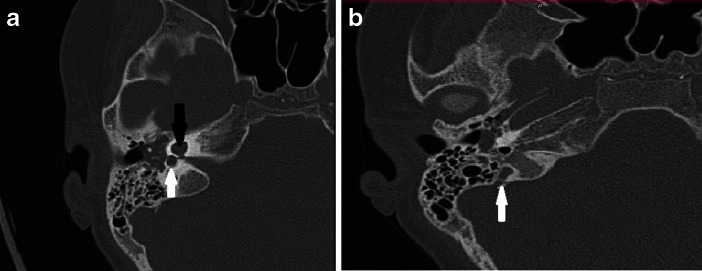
a. CT scan, temporal bone, axial view shows preserved basal turn but absence of the apical turns of the cochlea (black arrow), dilated vestibule (white arrow). b. CT scan, temporal bone, axial view shows enlarged vestibular aqueduct (white arrow)
